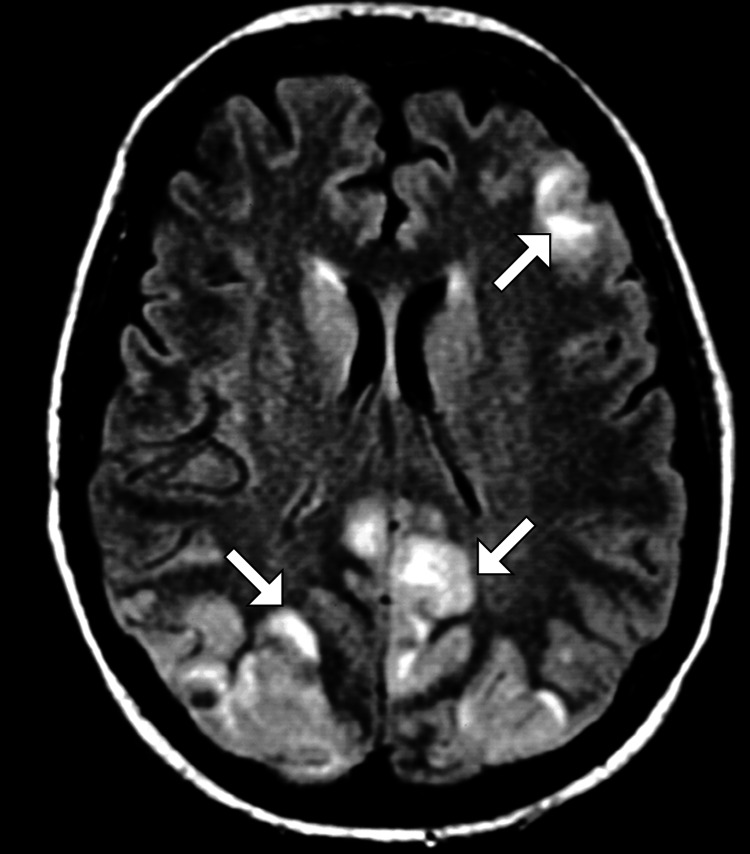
MRI of the brain An axial image of MRI of the brain demonstrates multifocal subcortical white matter with increased signal intensity (arrow) and associated vasogenic edema. MRI, Magnetic resonance imaging
